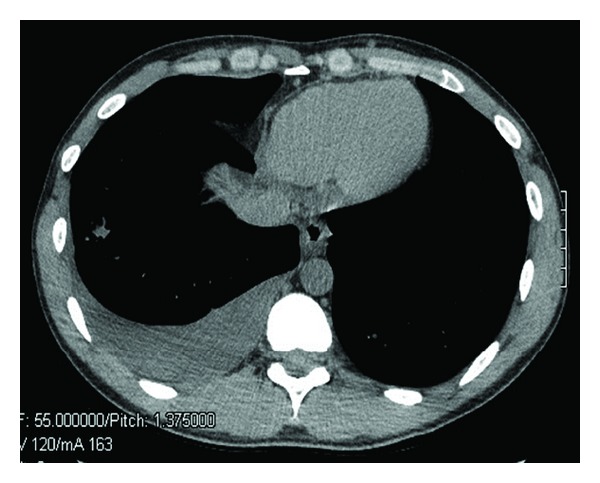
CT scan of the chest showing a 1.1 cm lobular opacity in the right lower lobe and a moderate right pleural effusion.
